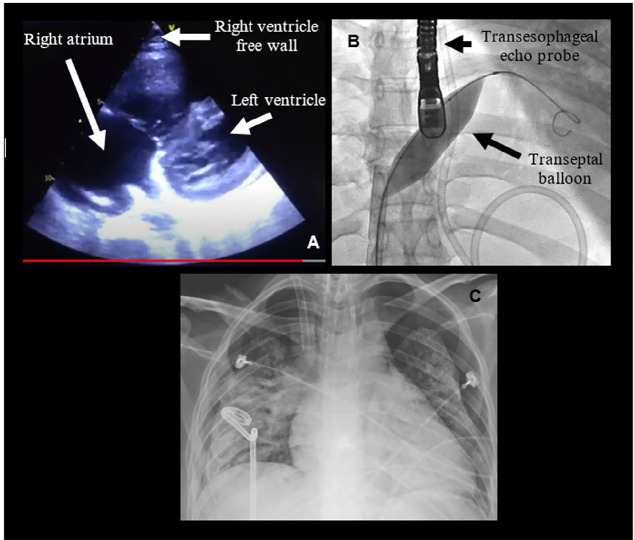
(A) Transthoracic echocardiography after the installation of severe
